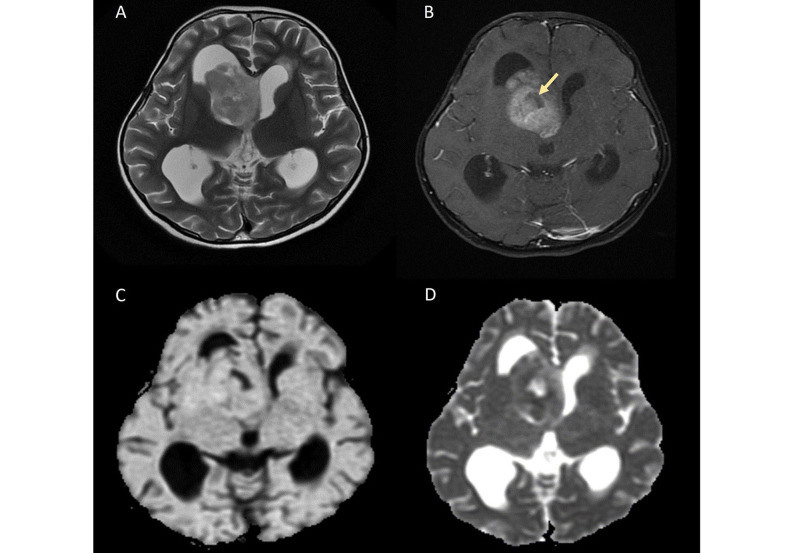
Intraventricular STE. A. Axial T2W image shows a heterogeneously hyperintense solid mass arising from the right lateral ventricle; B. axial contrast-enhanced image demonstrates heterogeneous enhancement of the mass with areas of necrosis (yellow arrow); C. on diffusion-weighted imaging (DWI) and D. apparent diffusion coefficient (ADC) map, the lesion shows patchy areas of restricted diffusion
